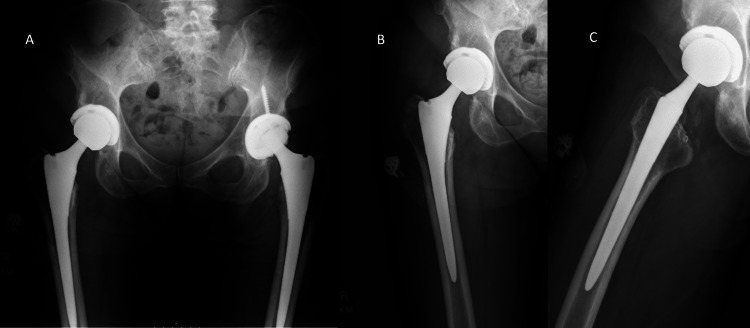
Postoperative anteroposterior (A) radiograph of the pelvis and anteroposterior (B) and lateral (C) radiograph of the hip showing a right total hip revision after bearing exchange
